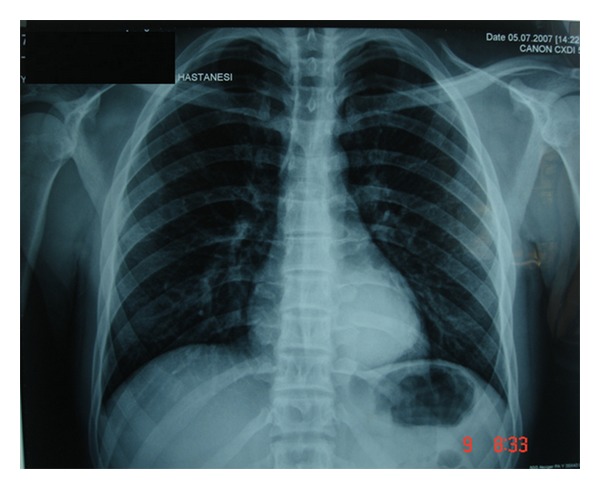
PA chest X-ray revealed a well-demarcated 6 cm peripheral opacity in the left lower lobe nearly by the diaphragm.
